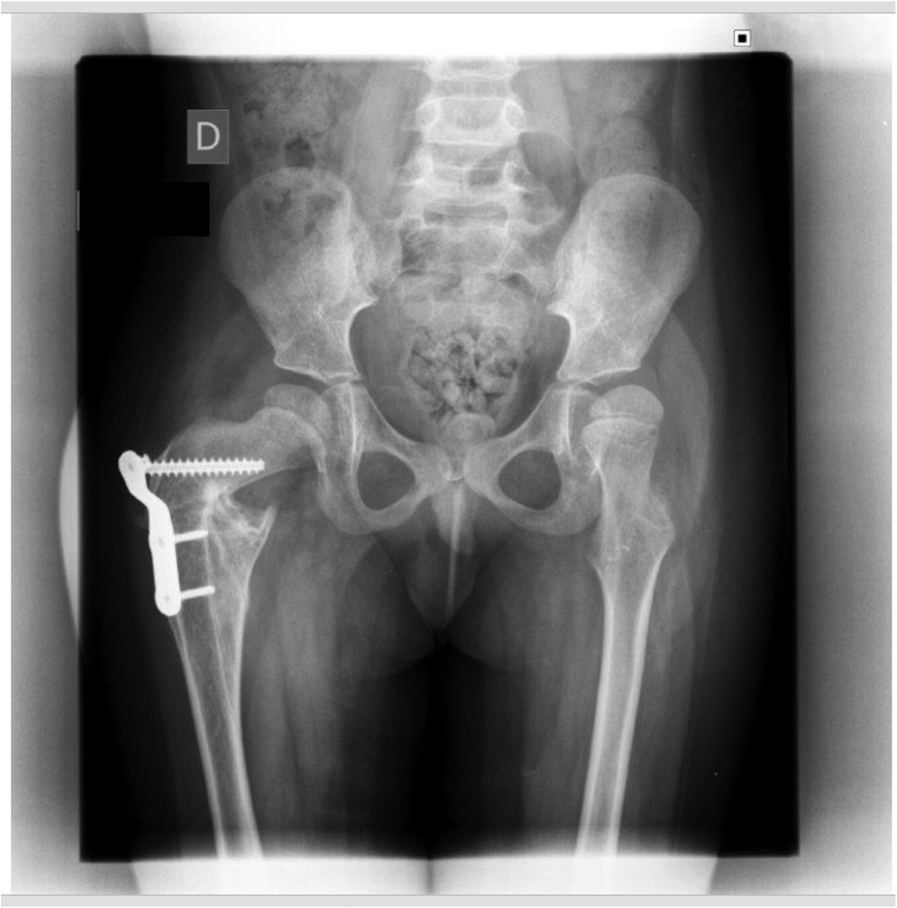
AP pelvis radiograph demonstrating another NGLY-1 deficient patient who has undergone proximal femoral osteotomy to treat neuromuscular hip dysplasia and hip subluxation
